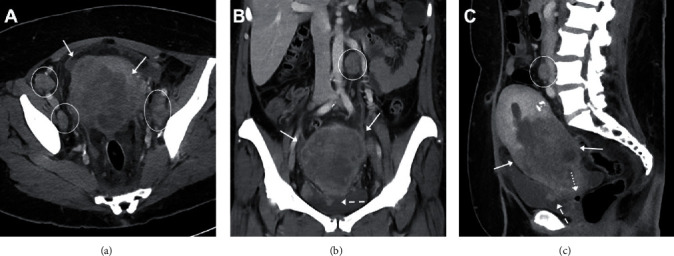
Axial (a), coronal (b), and sagittal (c) postcontrast CT images show a large heterogeneous mass centered within the lower uterus and cervix (solid arrows) which involves more than 50% of the myometrium and is enhancing less than the normal myometrium. There is local invasion to the urinary bladder (dotted arrows, (b, c)) and bilateral pelvic and retroperitoneal lymphadenopathy (circles/ellipses, (a–c)). There is no clear distinction with loss of the fat plane between the vagina and the rectum. Air focus within the vagina (dashed arrow, (c)) is suspicious for a rectovaginal fistula.
